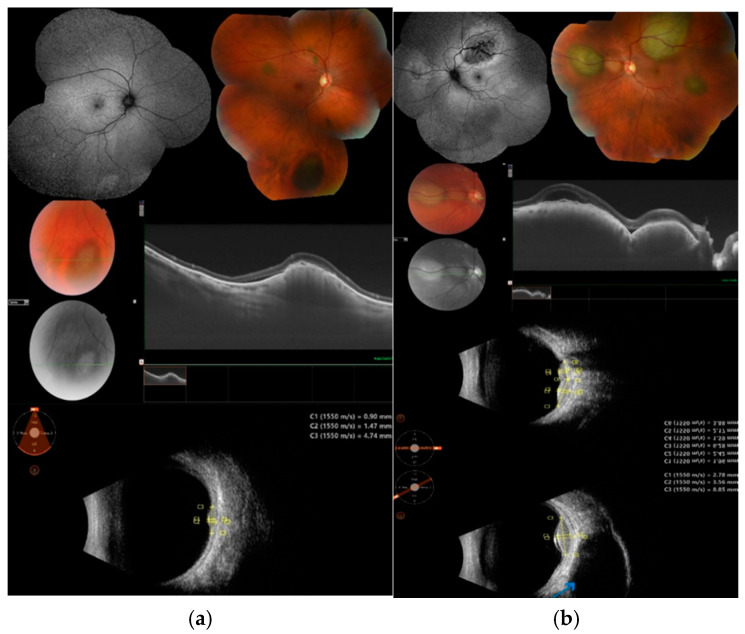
Multiple choroidal metastases in the right (a) and left (b) eyes of a 32-year-old woman with malignant melanoma of the skin, as seen on fundus autofluorescence, color fundus photography, OCT, and ultrasound. These findings are accompanied by a metastasis to the left orbit, visible on an ultrasound image as a hypoechoic lesion behind the eye wall (blue arrow).
